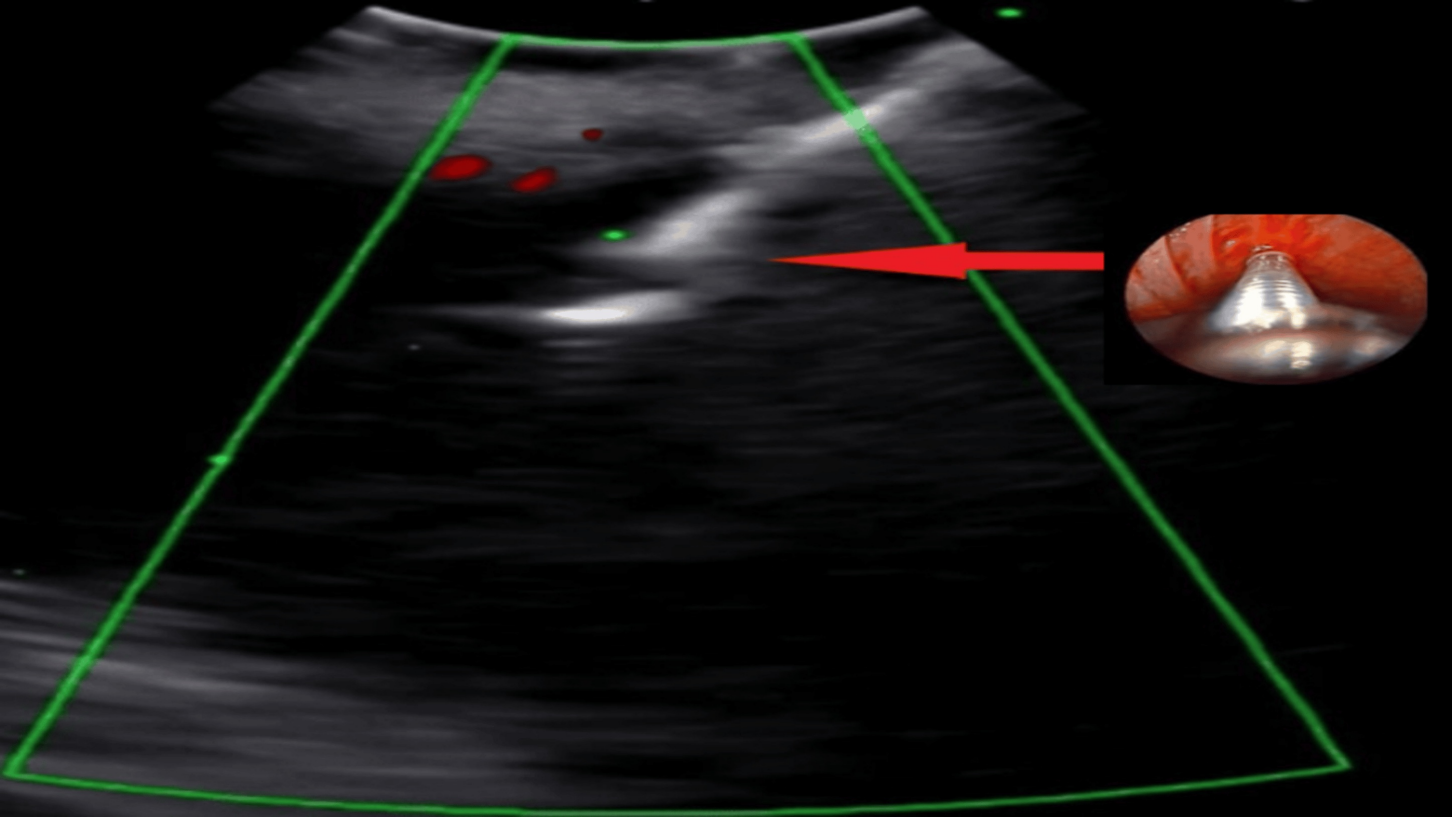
Linear EBUS TBNA from subcarinal LN The arrow points to endobronchial needle. EBUS: Endobronchial ultrasound; TBNA: Transbronchial needle aspiration; LN: Lymph node
